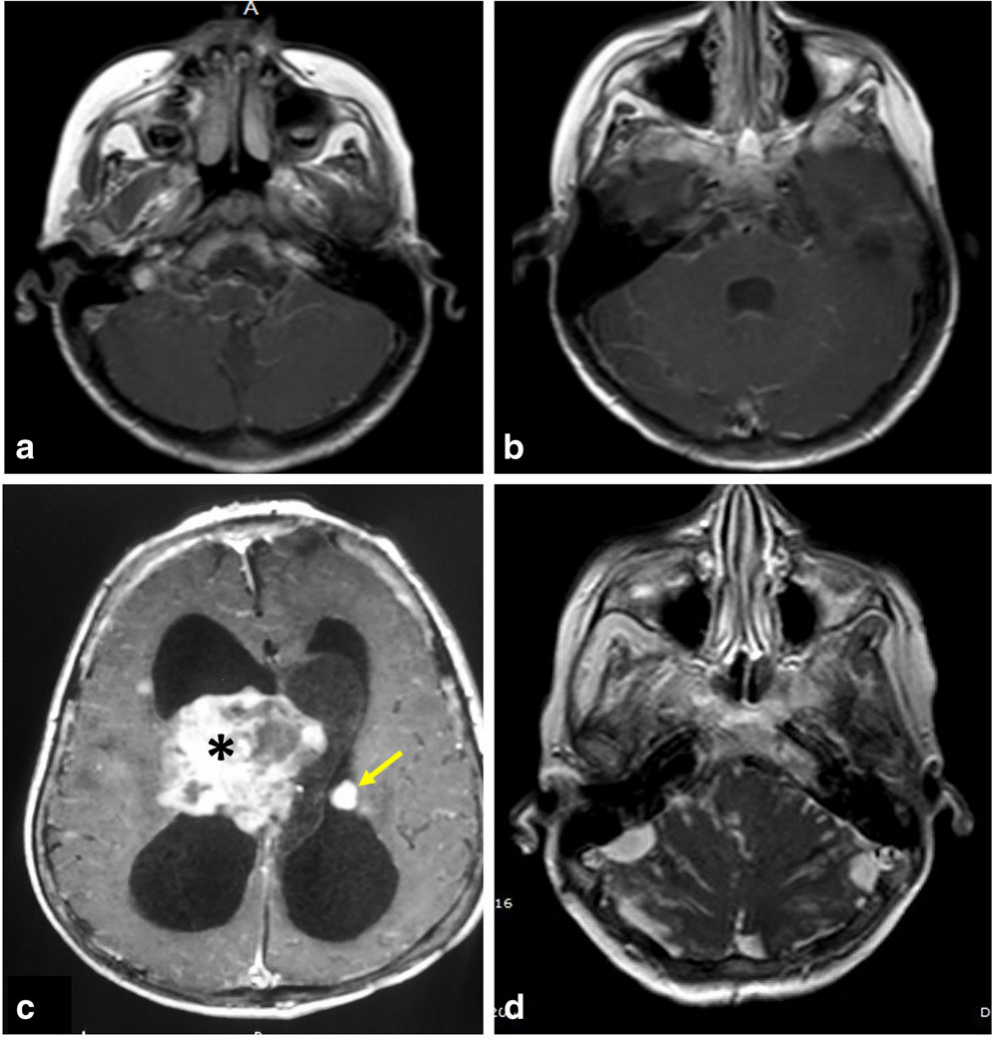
The value of post-contrast T1-WI in different cases with hydrocephalus. a, b MRI of a 16-year-old boy with hydrocephalus secondary to meningitis demonstrating smooth meningeal enhancement. c MRI of an 8-year-old girl with tuberous sclerosis demonstrating enhanced giant cell astrocystoma (asterisk) and subependymal nodule (arrow). d MRI of a 5-year-old girl with medulloblastoma showing nodular enhancement representing meningeal metastasis
